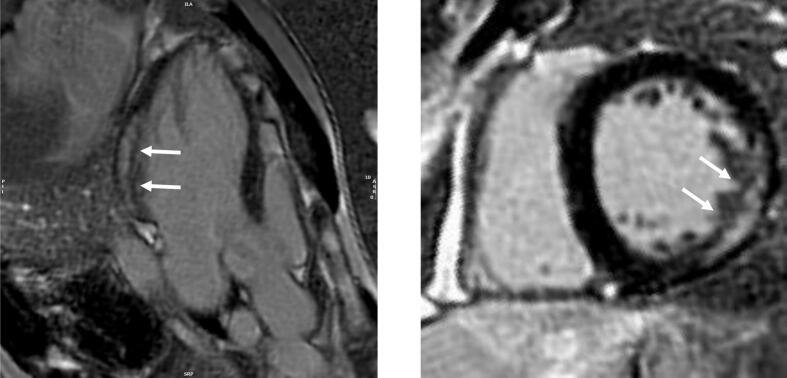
Cardiac MRI Fig. 4a-b. Steady state free precession cine imaging (4-chamber view; left panel and mid short axis, right panel), showing left ventricular myocardial thickness within normal limits (interventricular septum thickness = 10 mm, lateral wall thickness = 7 mm).c Native T1 mapping (mid short-axis slice) showing high native T1 values (ranging from 1100 ms to 1350 ms, suggesting high interstitial component secondary to fibrosis) with the exception of the anterior/anterolateral segments (white arrows), where native T1 values are low (920–930 ms, suggesting intramyocardial lipid accumulation).d-e. Phase sensitive inversion recovery late gadolinium enhancement imaging (4-chamber view, left panel, and mid short-axis view, right panel) showing dense intramyocardial enhancement in the basal-mid inferolateral segments (white arrows), indicating replacement fibrosis.
